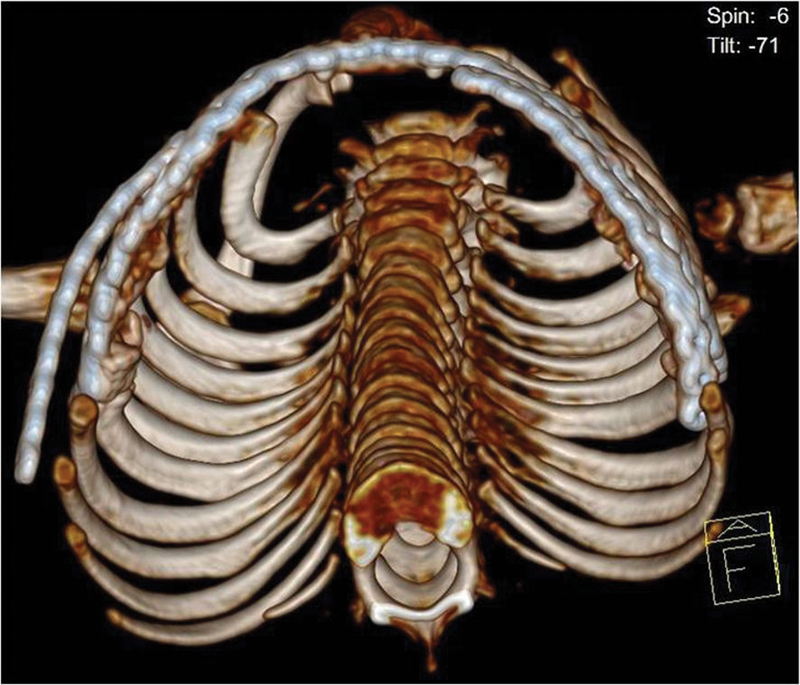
Three-dimensional reconstruction computed tomography scan of the chest after the first operation; axial view. Note the restored anterolateral contour of the chest.
